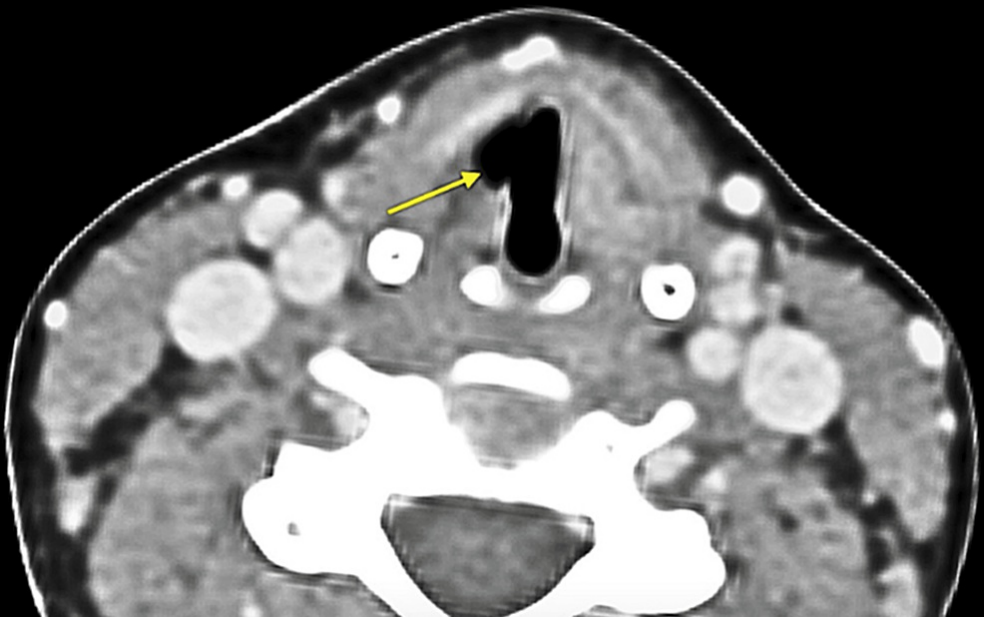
The Sail sign on axial CT sections at the level of the vocal cords Axial CT sections at the level of the vocal cords show dilation of the right laryngeal ventricle (arrow) and mild anteromedial displacement of the right arytenoid cartilage. The dilation of the ipsilateral laryngeal ventricle due to recurrent laryngeal nerve palsy resembles the sail of a ship and is known as the “Sail” sign.
